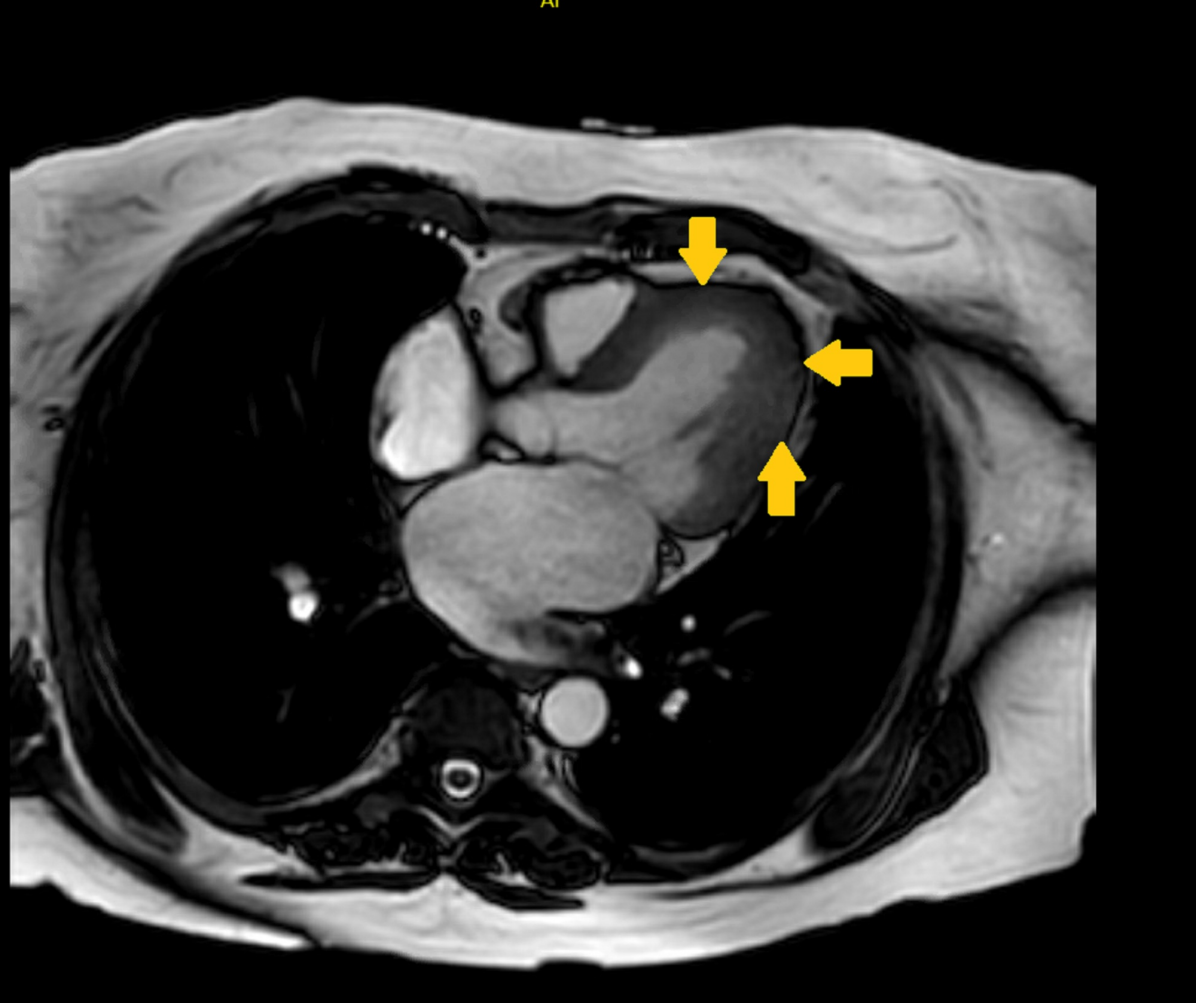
Cardiac MRI Cardiac MRI axial view showing low subendocardial signal in the left ventricular apical segment (arrows)
